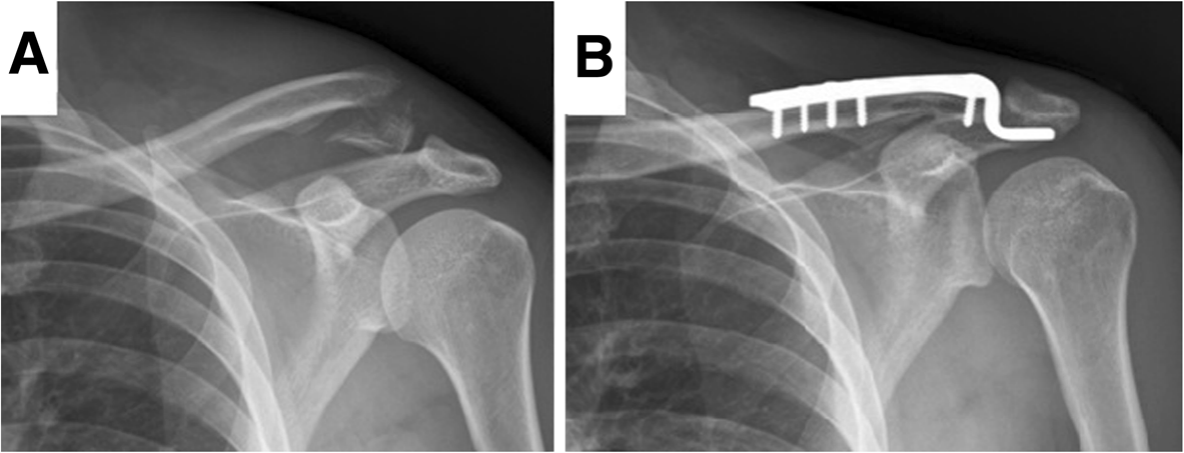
Stabilization by hook plate. (A) Preoperative radiography. (B) Postfixation radiography.
